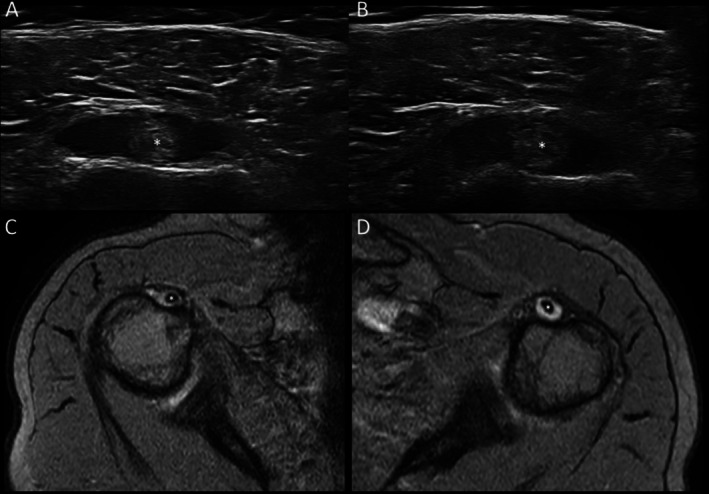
Polymyalgia rheumatica. An 80‐year‐old man with recent onset of inflammatory pain involving the shoulder and pelvic girdles, accompanied by a biological inflammatory syndrome. Transverse ultrasound (US) (A, right and B, left) and axial T2‐weighted gradient echo magnetic resonance images (MRI) (C, right and D, left) sequences of shoulders showing a representative example of bilateral long head of the biceps tenosynovitis. Notably, the widening of both tendon sheaths is due to anechoic (US) or hyperintense (MRI) signals, indicating an abnormal accumulation of synovial fluid. Asterisk indicates tendon of long head of the biceps.
